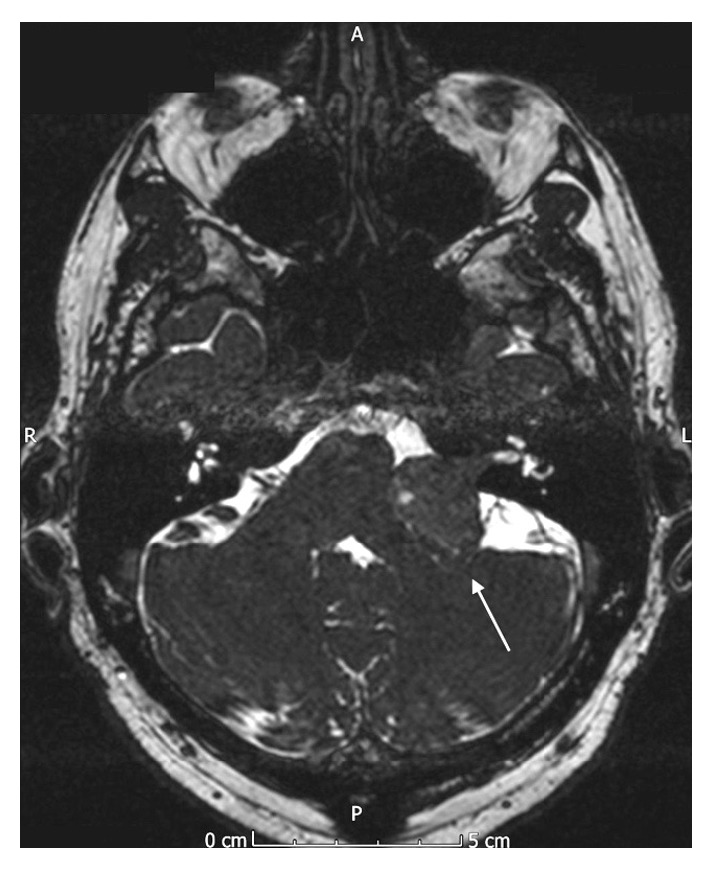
Axial MR image (T2 diffusion-weighted image) showing the vestibular schwannoma affecting the left vestibulocochlear nerve.
